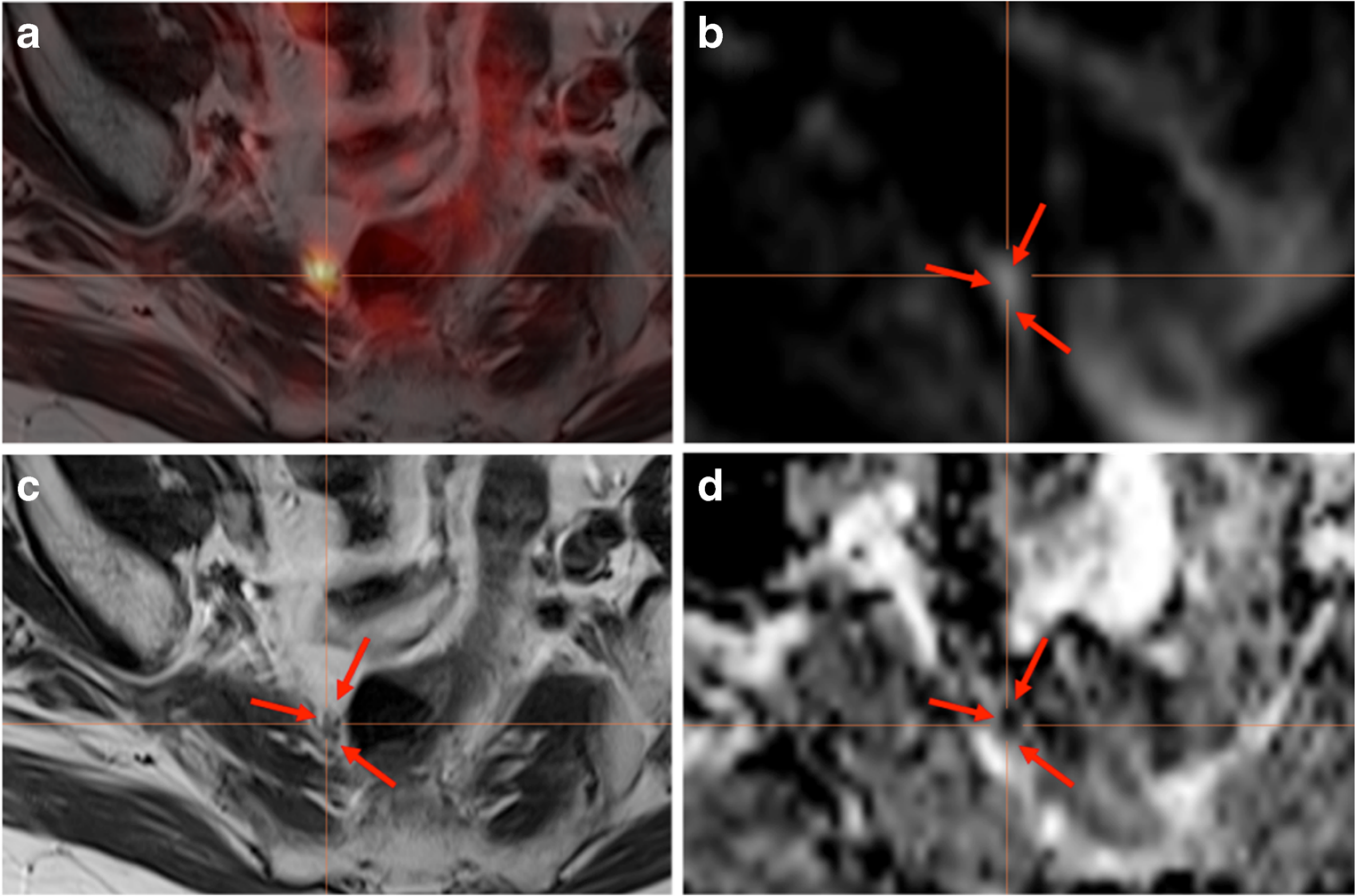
Additional diagnostic value of simultaneous PET/MRI: 77 y/o, post-RPE + RT, pT3a pN1 Gleason 9, PSA at PET/MRI: 1.35 ng/ml: Confirmation of positive [^68^Ga]-PSMA^HBED-CC^ conjugate 11 uptake in a pararectal lymph node (a; ca. 5 mm), which is difficult to detect on T2w turbo spin echo sequences (c), but has a significant diffusion restriction on DWI b600 images (b) and a low apparent diffusion coefficient (d), which confirms high cell density in this area. Androgen deprivation therapy was applied. The actual PSA serum level is still <0.2 ng/ml
